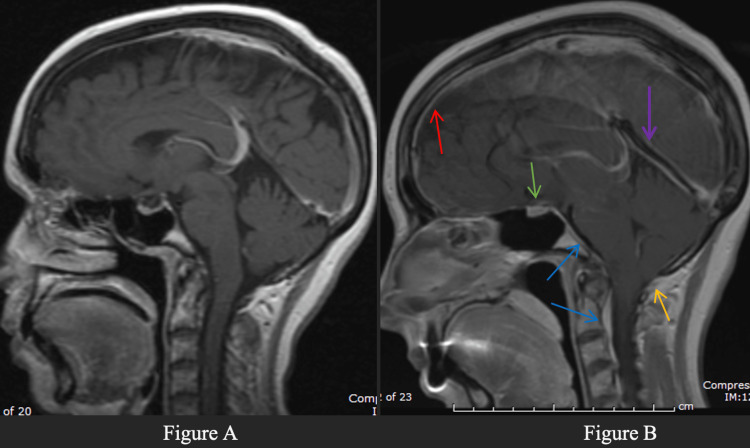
Normal MRI vs MRI with appreciated SIH. A: This is a normal MRI brain scan (left, sagittal postcontrast T1W pulse sequence) in same patient done 7 years ago. B: Typical findings of intracranial hypotension seen in current study shown above. This MRI was done 7 years from the previous scan after patient presented with multiple symptoms of headache, nausea, and left sided worsening weakness. Diffuse dural thickening and enhancement (red arrow). Venous engorgement along clivus and epidural veins, and a flattened pons (blue arrows). Lower position of cerebellar tonsils (yellow arrow). Pituitary stalk is curving posteriorly due to brain stem descent (green arrow). Congestion of the straight sinus (purple arrow). SIH, spontaneous intracranial hypotension
